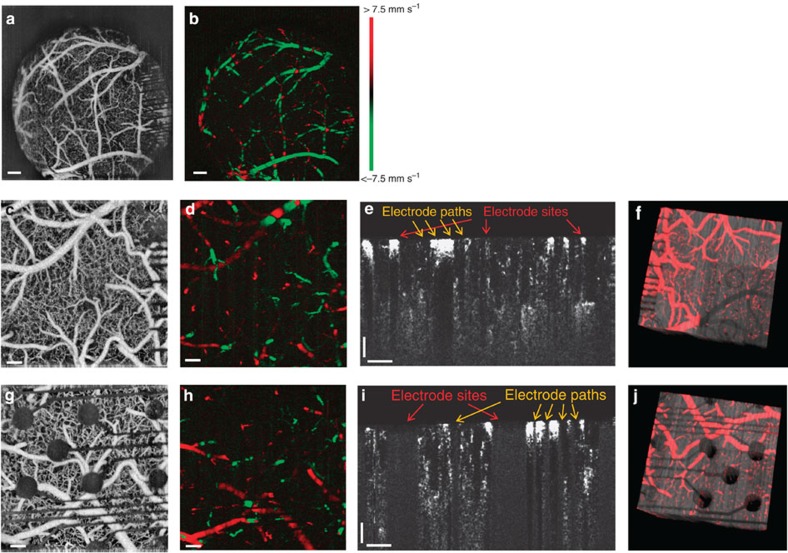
Optical coherence tomography through CLEAR and platinum devices. (a,c) Maximum intensity projection (MIP) of OCT angiogram showing cortical vasculature visible through the CLEAR micro-ECoG device (FOV 2.8 × 2.8 mm^2^ and 1.1 × 1.1 mm^2^, respectively). (b,d) Doppler blood flow velocity image showing the directionality of blood flowing through the vasculature below the CLEAR device (FOV 2.8 × 2.8 mm^2^ and 1.1 × 1.1 mm^2^). Red colour represents blood flowing towards the lens and green colour represents blood flowing away. (e) Cross-sectional angiogram after contrast enhancement and de-shadowing (CLEAR device). (f) 3D visualization of the vasculature (red colour) overlaid on the structural data (grey) (CLEAR device). (g) MIP of angiogram through a platinum micro-ECoG device. (h) Corresponding Doppler blood flow velocity measurements. (i) Corresponding cross-sectional angiogram. (j) Corresponding 3D visualization of vessel structure for a micro-ECoG array with platinum electrode sites. Scale bars, 200 μm (a,b), 100 μm (c–i).
